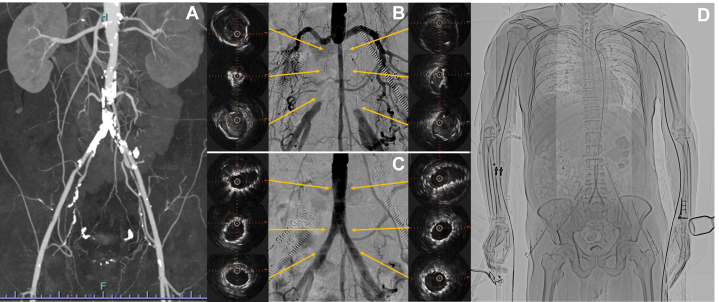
Overview of Dual Transradial Intervention for Aortoiliac Occlusion (A) CT angiography, (B) preprocedural angiography and IVUS findings after guidewire passage, (C) postprocedural angiography and final IVUS findings, and (D) dual transradial approach. CT = computed tomography; IVUS = intravascular ultrasound.
